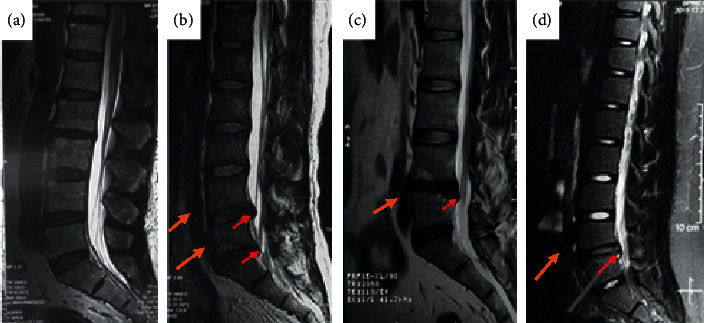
MRI of patients with LDH. (a) shows an MRI image of lumbar disc of a normal human body; (b) shows an MRI image of a male patient aged 42 years with L4/5 disc herniation; (c) shows an MRI image of L3/4 disc herniation for a female patient aged 39 years; and (d) shows an MRI image of L5/S1 right disc herniation for a male patient aged 64 years.
